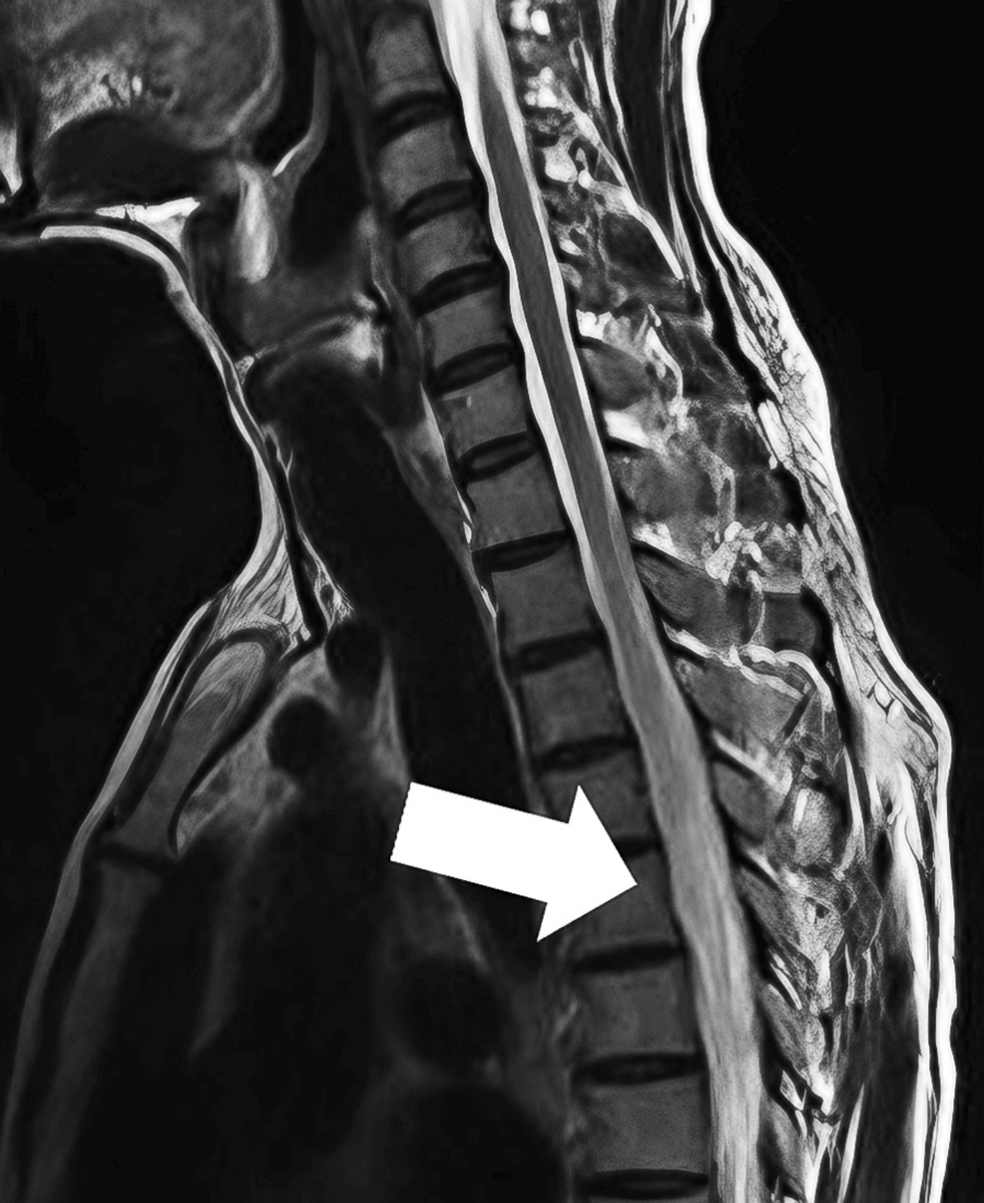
The spinal MRI. The MRI revealed an intramedullary T2 hyperintense signal abnormality in the central gray matter from the C5 to T4 levels of the spinal cord (as shown by the arrow). MRI: magnetic resonance imaging
